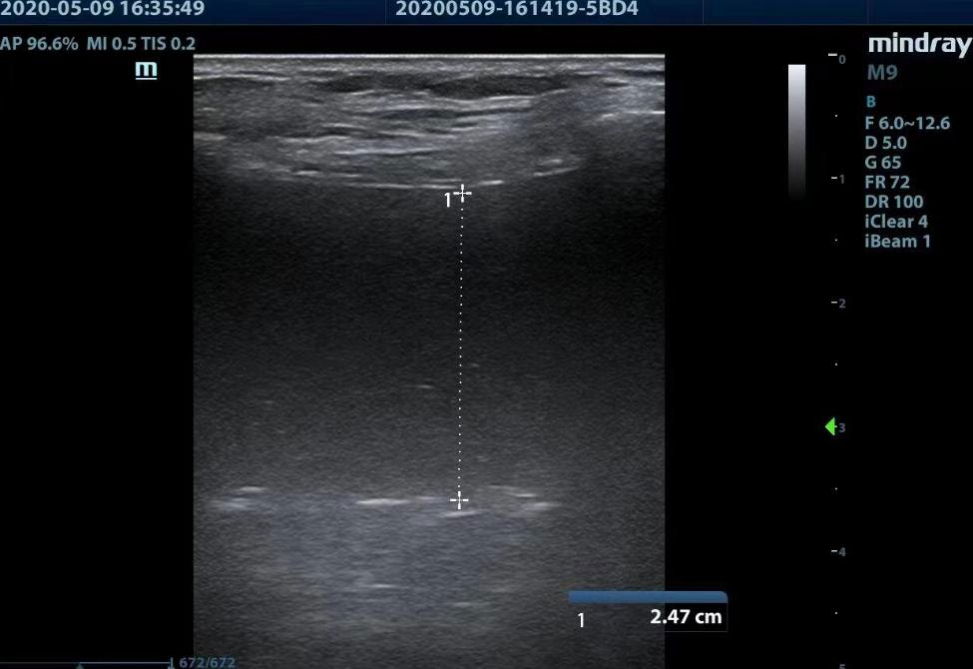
Ultrasonography demonstrating the presence of pleural effusion in the right midaxillary line
